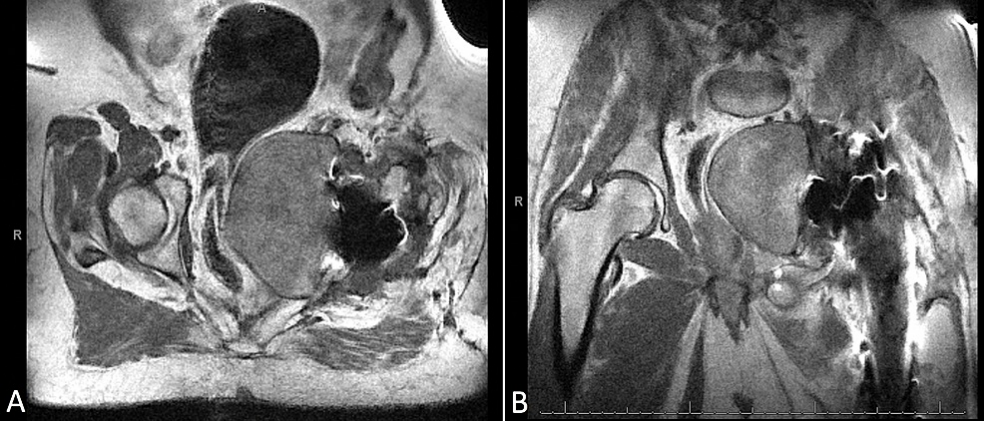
Preoperative MRI images. Preoperative axial MRI (a) demonstrating displacement of the bladder as well as compression on the rectum due to large pseudotumor associated with the left hip. Coronal MRI (b) demonstrating large cystic mass displacing iliac vessels and the rectum.
